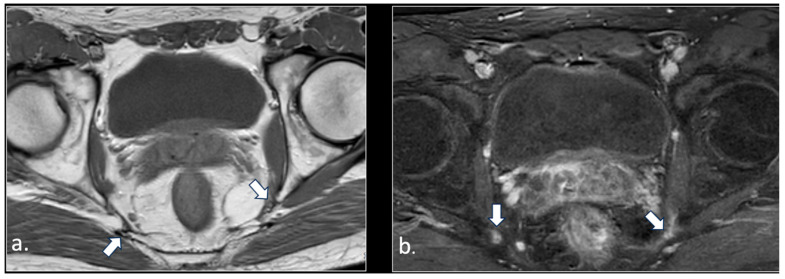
A 32-year-old man with relapsing pudendalgia after decompressive surgery on both pudendal nerves. (a) Axial T1-weighted image, obtained at the level of the ischial spine, shows fibrosis along the extra-pelvic course of both pudendal nerves (arrows). (b) Axial T1-weighted enhanced subtracted scan demonstrates enhancement along the course of the nerves (arrows).
